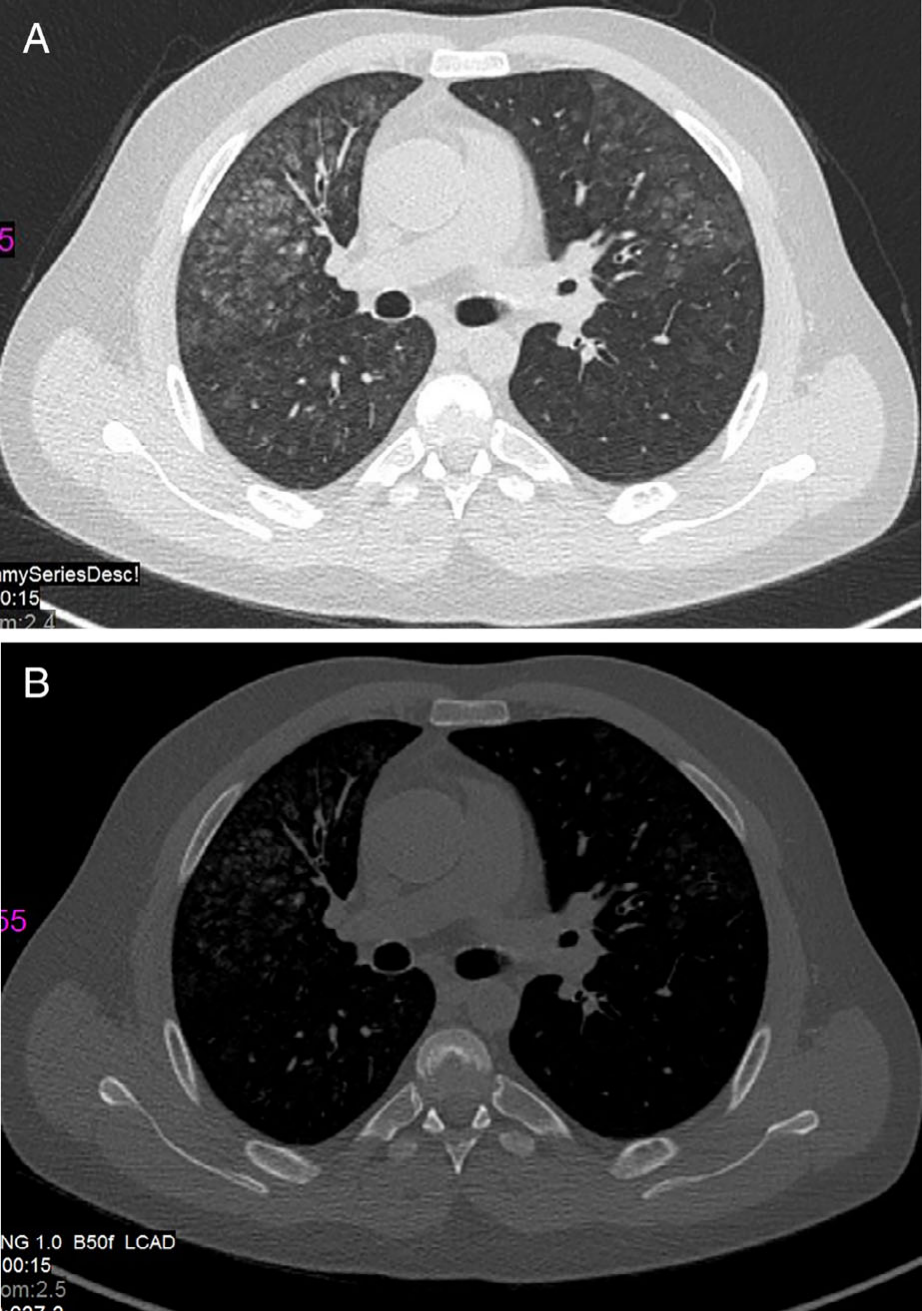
Case 1: A 40‐year‐old male with chronic renal failureand diagnosis of metastatic pulmonary calcification. (A) High‐resolution computed tomography shows upper lungs predominant fluffy‐appearing centrilobular ground‐glass opacities. (B) Bone windows show no apparent calcifications
